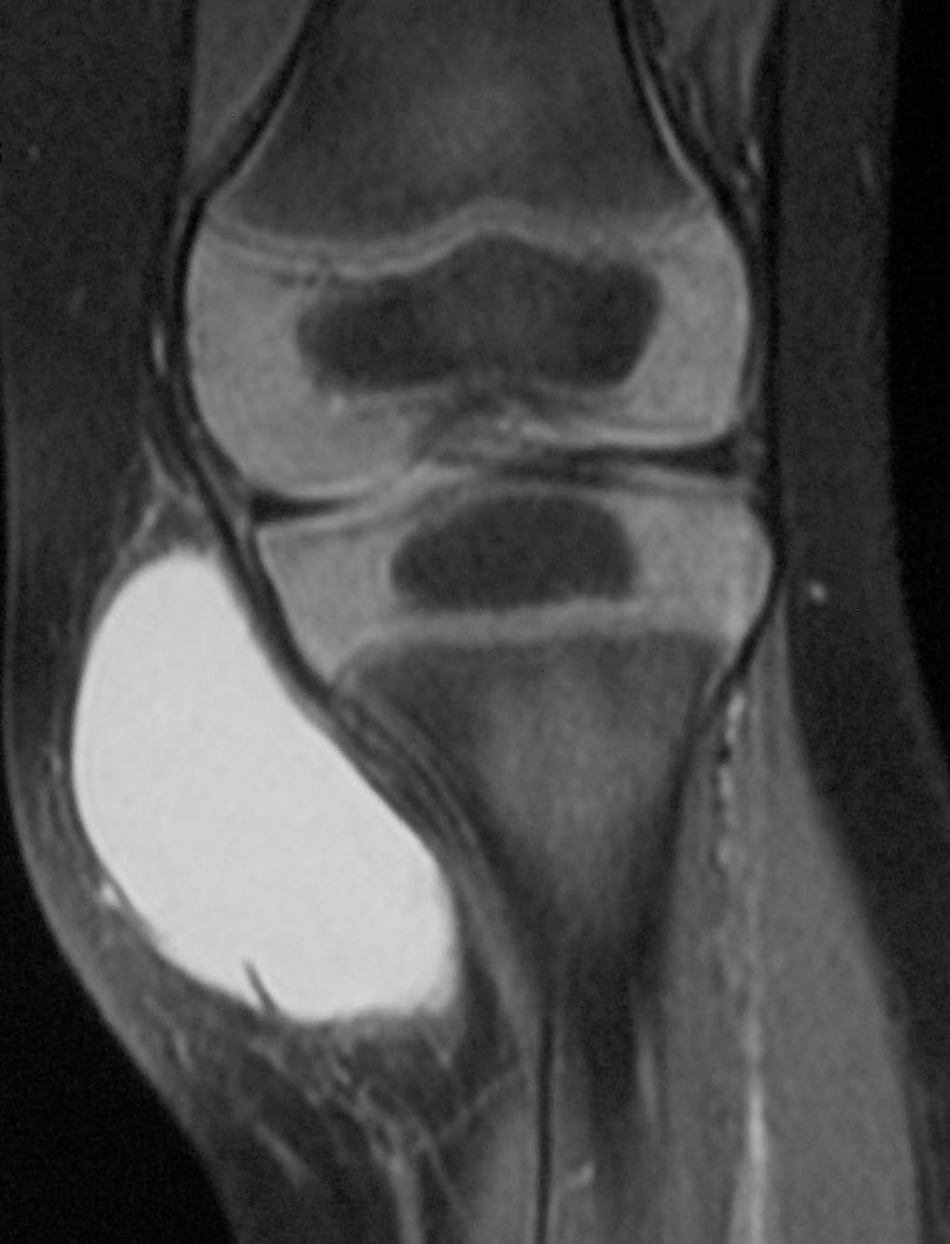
Case 1- MRI defines the margins of the tumor.
